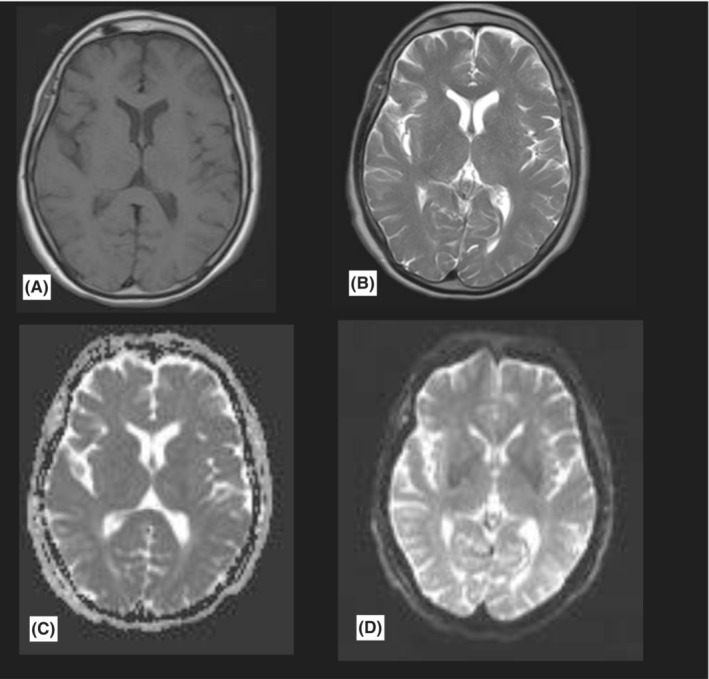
Brain MRI in serial sequences shows no acute intracranial lesions. A = T1, B = T2, C and D = perfusion‐diffusion sequences.
